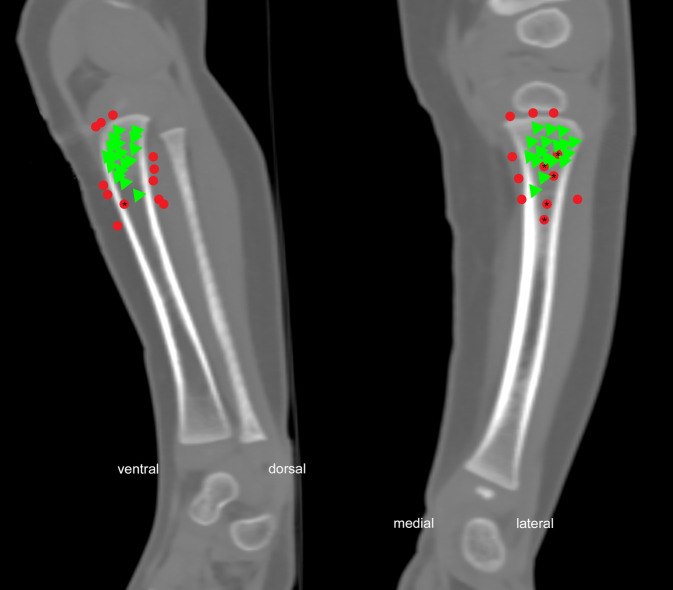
Darstellung aller 29 Punktionen in einem koronaren und sagittalen CT-Schnitt. *Rote Punkte* repräsentieren Fehllagen, *grüne Dreiecke* korrekte Lagen, jeweils bezogen auf das Ende des Stichkanals. Die mit einem *Asterisk* gekennzeichneten Fehlpunktionen liegen aufgrund der Limitation der Darstellung nur scheinbar korrekt, was durch die Visualisierung in der zweiten Ebene offenbar wird
